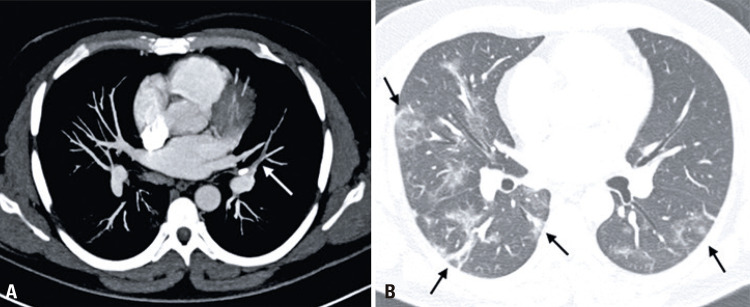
Computed tomography pulmonary angiography (41-year-old patient). (A) Axial mediastinal window shows lingular segmental pulmonary embolism (white arrows). (B) Axial pulmonary window reveals multiple ground-glass opacities, thickened interlobular septae, and parenchymal bands with bilateral and mainly peripherally distribution, findings consistent with COVID-19 pneumonia
